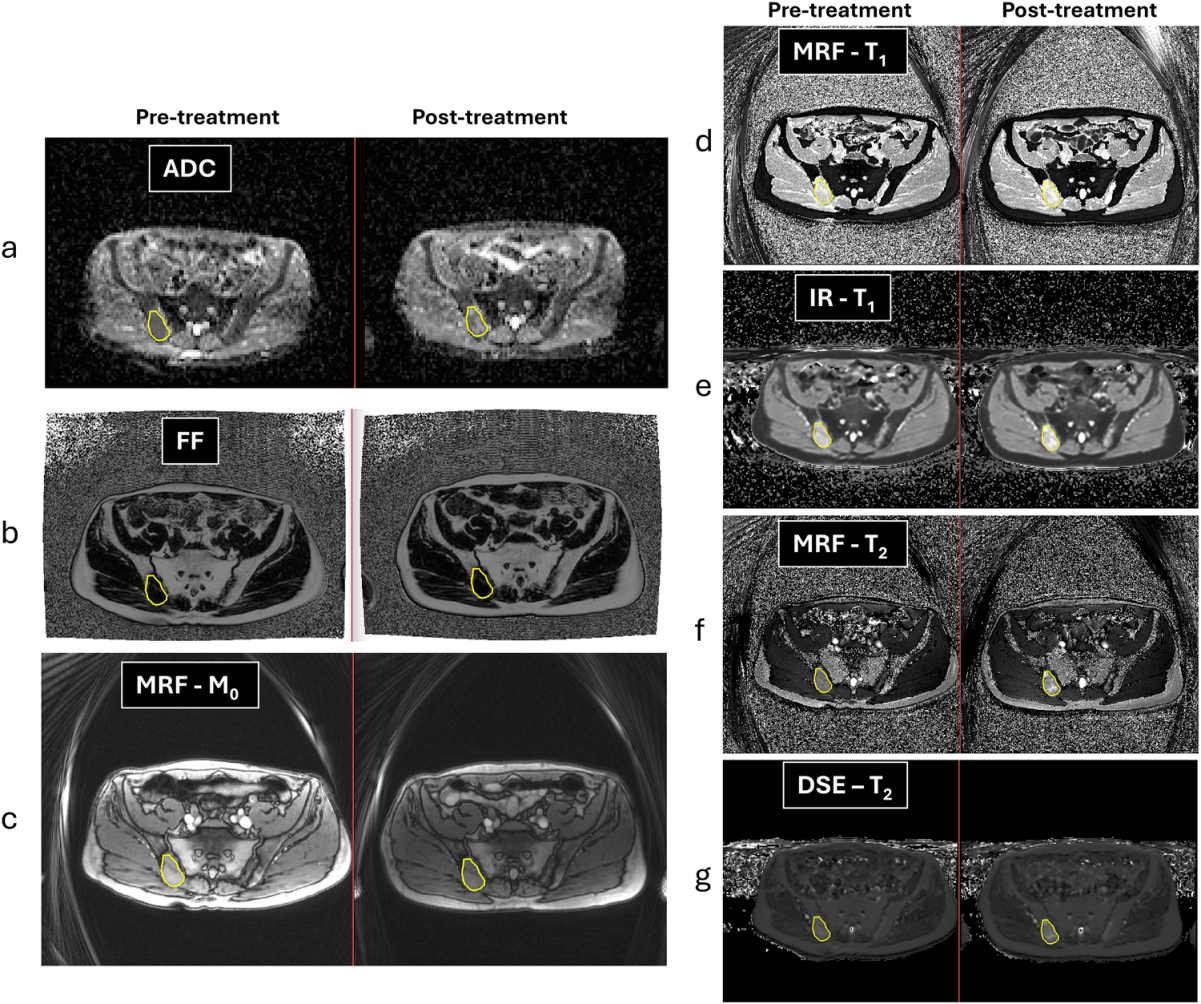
Pre- and post-treatment (11 weeks after start of new treatment line) images from a 66-year-old patient with an active lesion in the right iliac bone (ROIs drawn on the MRF M_0_ image). Matched parametric maps derived from DWI (a ADC map) and Dixon (b FF map) acquisitions visually helped to differentiate between normal and metastatic bone. Image contrast display for each type of measurement (c–g) was kept fixed between the two visits. Note that increases in T_1_ and T_2_ values after treatment were observed with both MRF and conventional MRI methods
